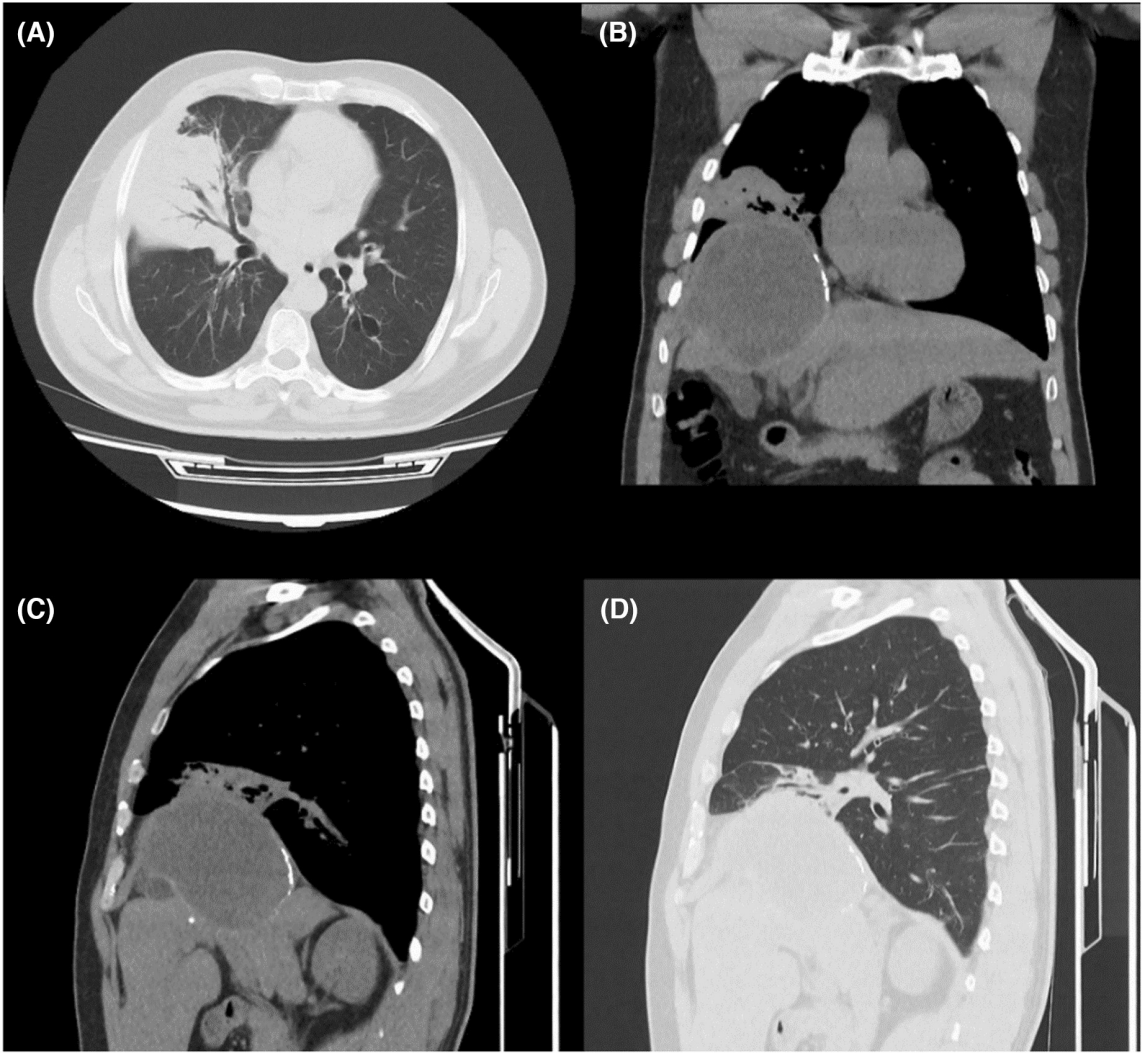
Preoperative chest computed tomography scan of a 48‐year‐old man with bronchobiliary fistula; (A) axial, (B) coronal, and (C), (D) sagittal views.
